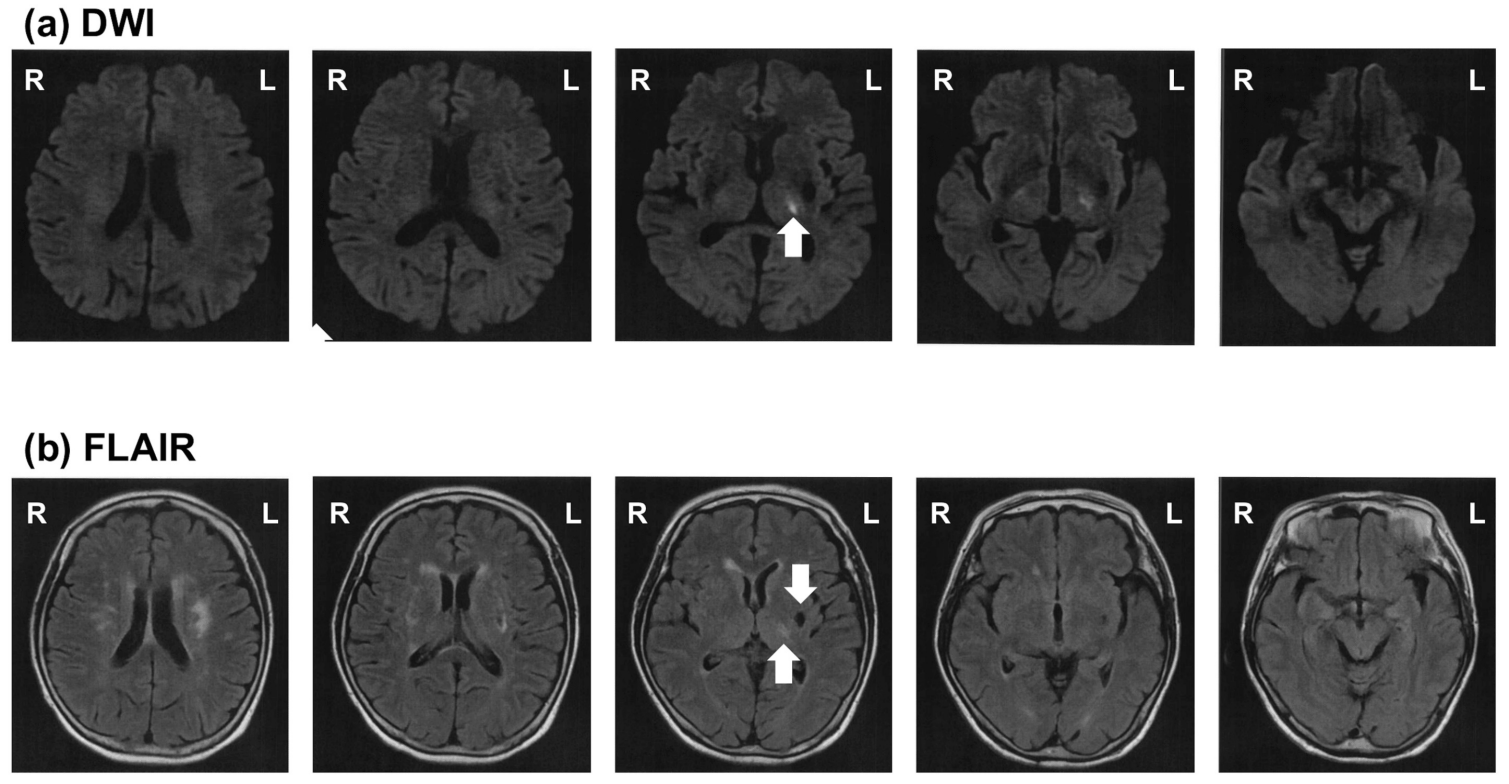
Day 1 brain MRI findings (a) Diffusion-weighted imaging (DWI) and (b) fluid-attenuated inversion recovery (FLAIR) show a high-signal lesion in the lateral part of the left thalamus (arrows). An old lacunar infarction is visible in the left basal ganglia. Axial FLAIR images were reconstructed with a slice thickness of 6.0 mm, a slice range of 7.5 mm, and an in-plane pixel size of 0.43 × 0.43 mm; the DWI sequence used the same slice thickness (6.0 mm) and slice range (7.5 mm) with an in-plane pixel size of 0.86 × 0.86 mm.
